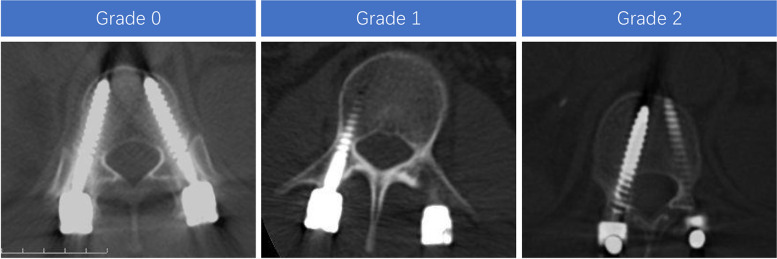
Representative examples of CT scans demonstrating the different grades of facet joints violation
